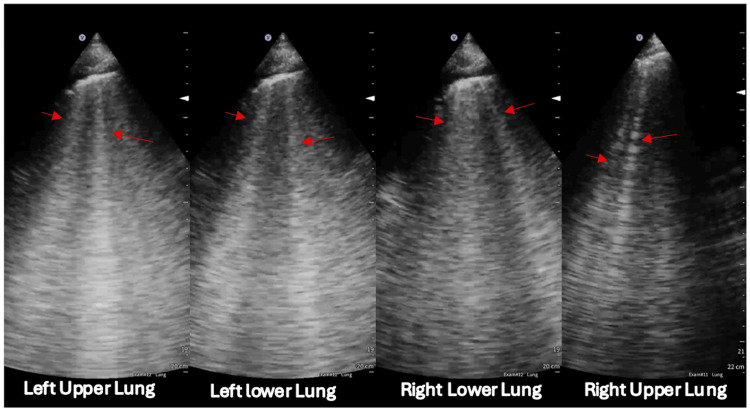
Lung Ultrasound Examination Lung ultrasound images obtained from the left upper, left lower, right lower, and right upper lung zones demonstrate multiple confluent vertical hyperechoic artifacts (B-lines) arising from the pleural line and extending to the bottom of the screen without fading (red arrows). Findings are present bilaterally across multiple lung fields most consistent with acute pulmonary edema.
